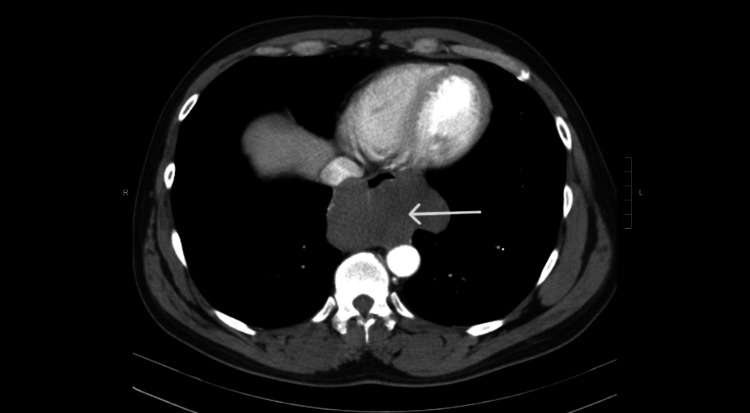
Contrast-enhanced CT abdomen demonstrating esophageal leiomyoma (white arrow) CT: computed tomography
